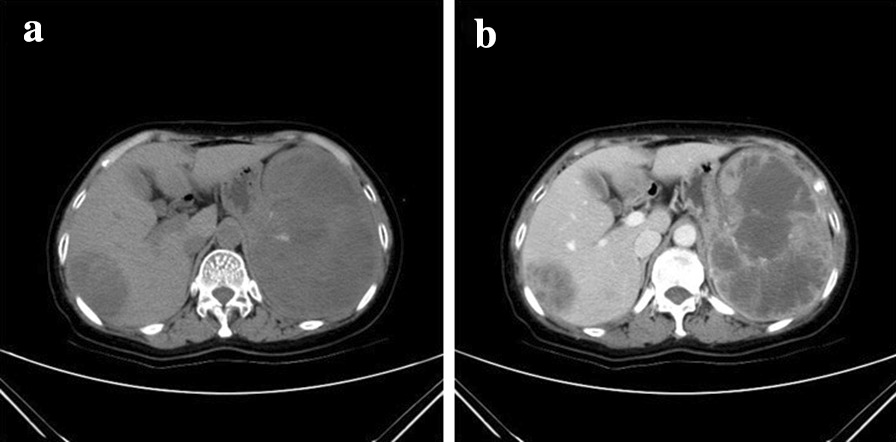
A 57-year-old female patient with multiple inflammatory pseudotumor-like follicular dendritic cell sarcomas in the liver and spleen. a The plain computed tomography scan reveals round-like hypodense masses with uneven densities in the spleen and liver. b After contrast enhancement, the masses show a honeycomb structure, and no enhancement can be seen in the central necrotic area
